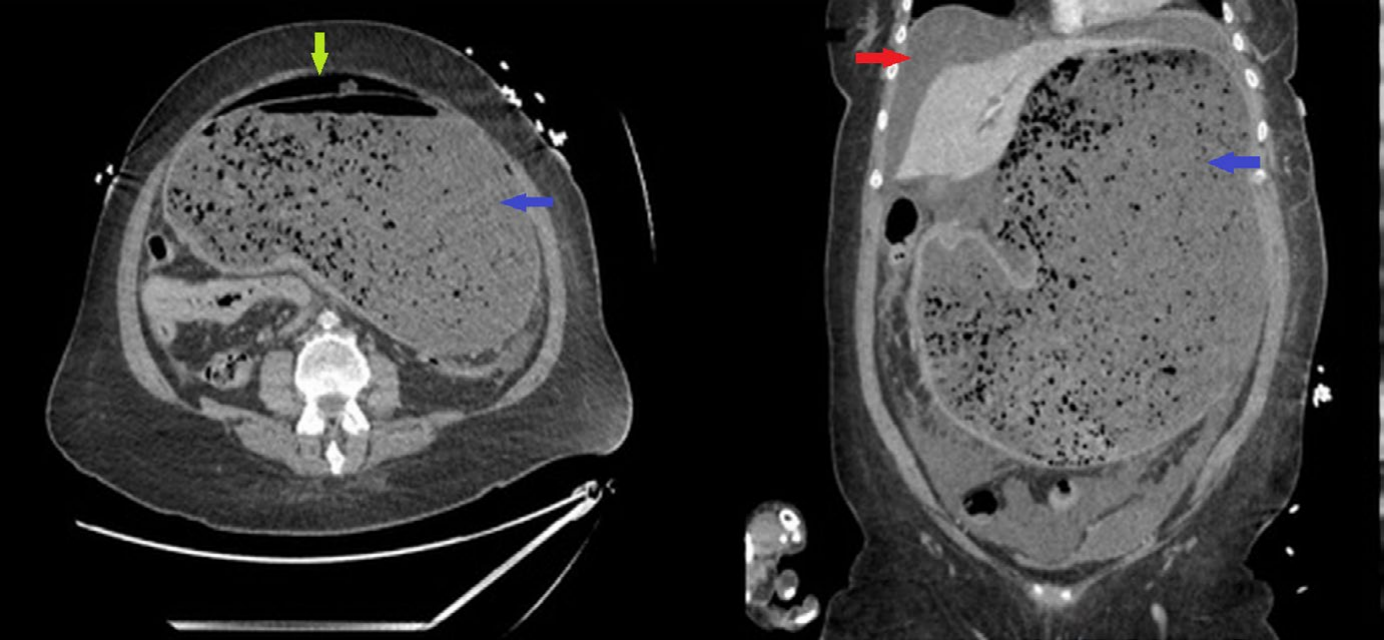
Computed tomography of the abdomen and pelvis (axial and coronal view) showing massive dilatation of the stomach containing food debris (blue arrow) with free intraperitoneal air (green arrow) and fluid (red arrow)
